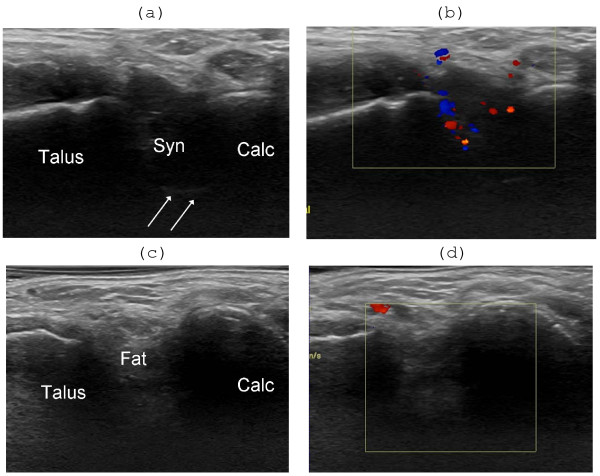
Effect of US-guided steroid injection in the antero-lateral recess of the posterior subtalar joint . Lateral oblique longitudinal scanning planes. (A) Before injection there is an enlarged hypoechoic antero-lateral recess (Syn), with distinct deep margins (arrows), bulging into the hyperechoic fat of the tarsal sinus between talus and calcaneus (Calc), and (B) synovial hyperemia at color Doppler examination. (C) One week after injection the recess is not visualized (D) and there is complete regression of synovial hyperemia.
